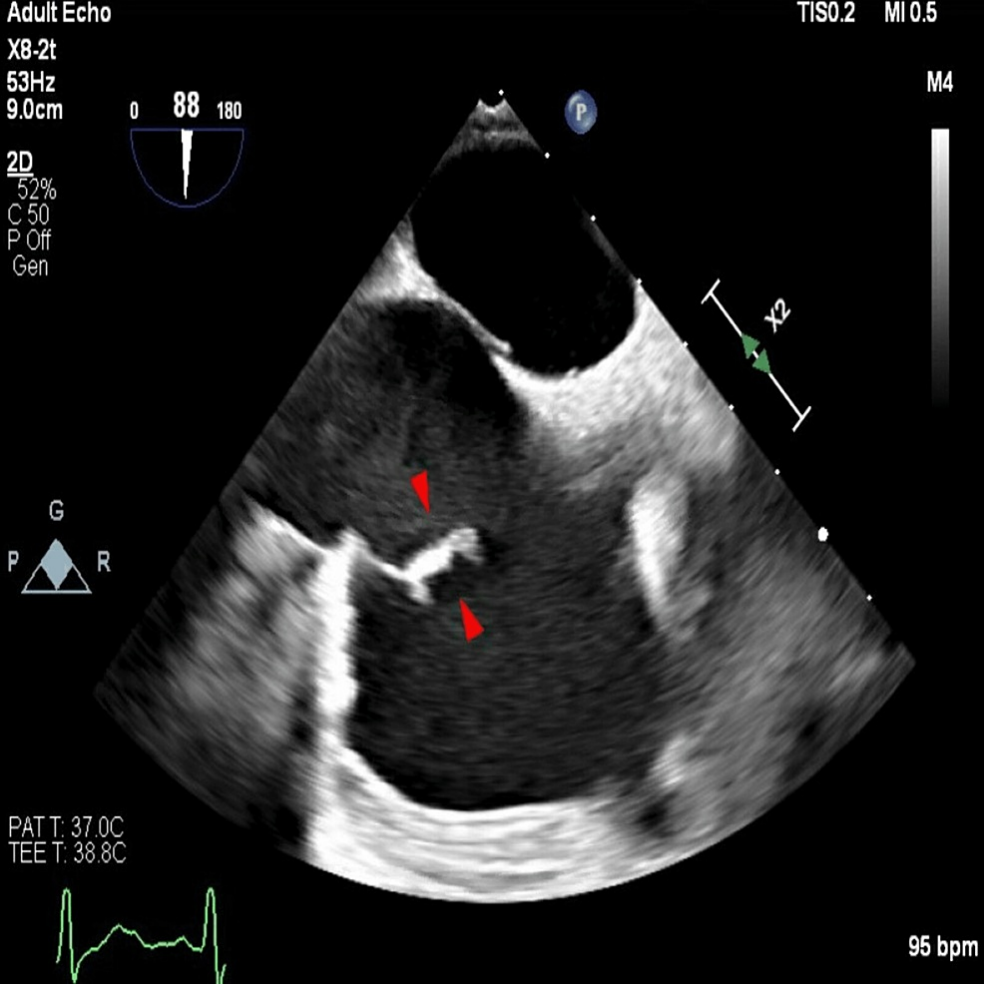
TEE showing a thickened eustachian valve within the right atrium visible with apparent fibrinous aggregate or vegetation, suspicious for endocarditis TEE: transesophageal echocardiogram
